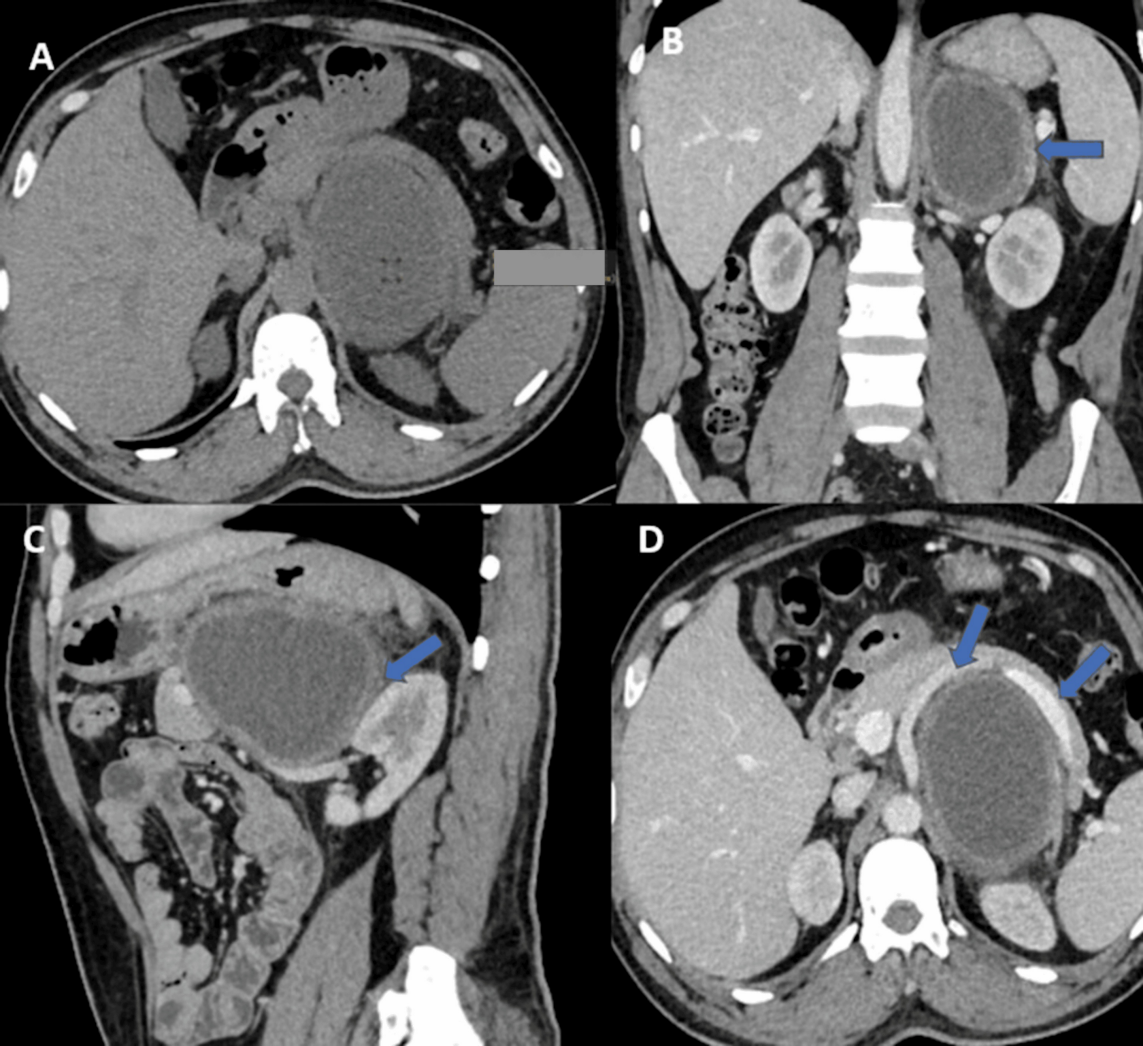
CT images of the patient (A) Non-contrast, axial image shows a fluid attenuation lesion in the left suprarenal location; (B) post-contrast, coronal CT image showing a cystic lesion with peripheral wall enhancement (arrow); (C) post-contrast, sagittal CT image showing the lesion is superior to the left kidney with maintained fat planes; (D) post-contrast, axial CT image showing anterior displacement of the left renal vessels by the lesion (arrows). CT: computed tomography
